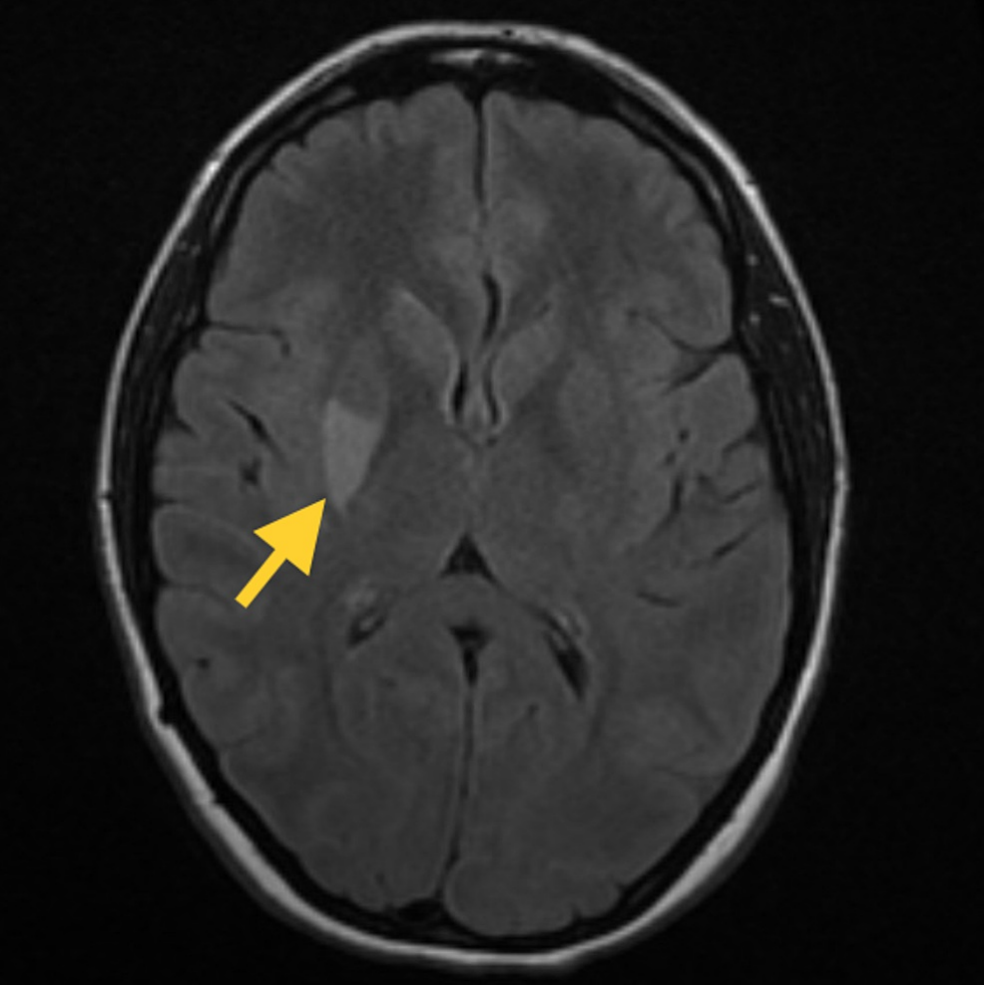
MRI Axial T2 FLAIR demonstrating right insular CVA (yellow arrow) MRI: magnetic resonance imaging; FLAIR: fluid-attenuated inversion recovery; CVA: cerebrovascular accident
